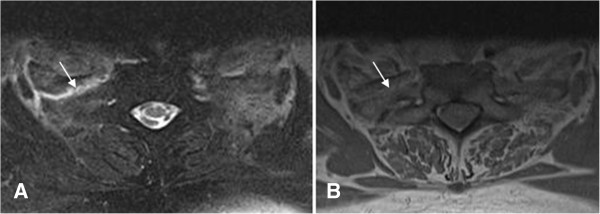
Cervical spine magnetic resonance imaging MRI. A T2 weighted image (A) reveals a displaced fracture at the proximal right first rib with increased signal intensity at the fracture site (white arrow). Image (B) reveals the displaced fracture at the proximal right first rib with isointense signal at the fracture site (white arrow). These findings are indicative of edema and hemorrhage.
